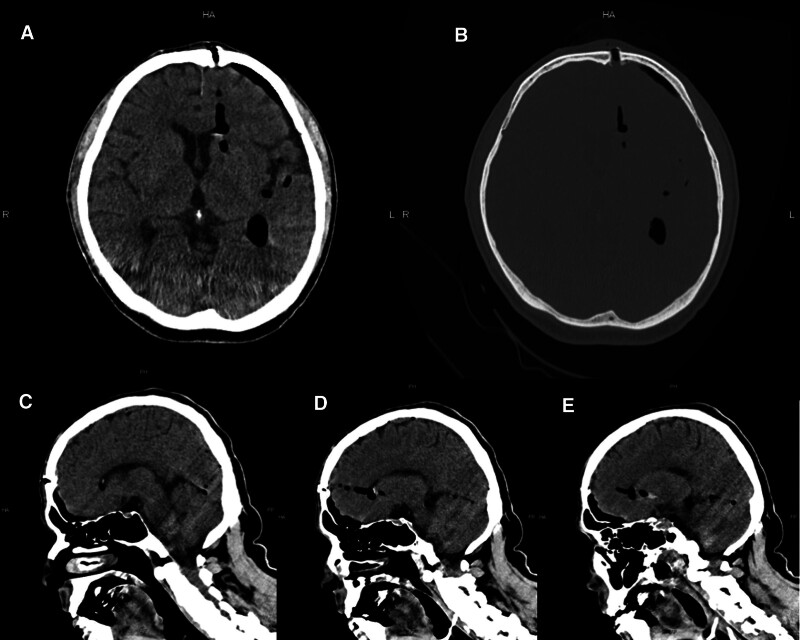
CT images of the head were obtained at the time of arrival at the hospital. Axial images demonstrate a frontal bone defect slightly to the left of the midline, and the route taken by the gimlet from the left frontal lobe through the anterior horn of the lateral ventricle, to the head of the caudate nucleus, putamen, and globus pallidus. A small amount of hemorrhage and air are present in the lateral ventricle and in the subarachnoid and subdural spaces (A and B). Sagittal reconstructions show the route of the gimlet through the frontal lobe, anterior horn of the lateral ventricle, head of the caudate nucleus, left putamen, and globus pallidus (C–E).
